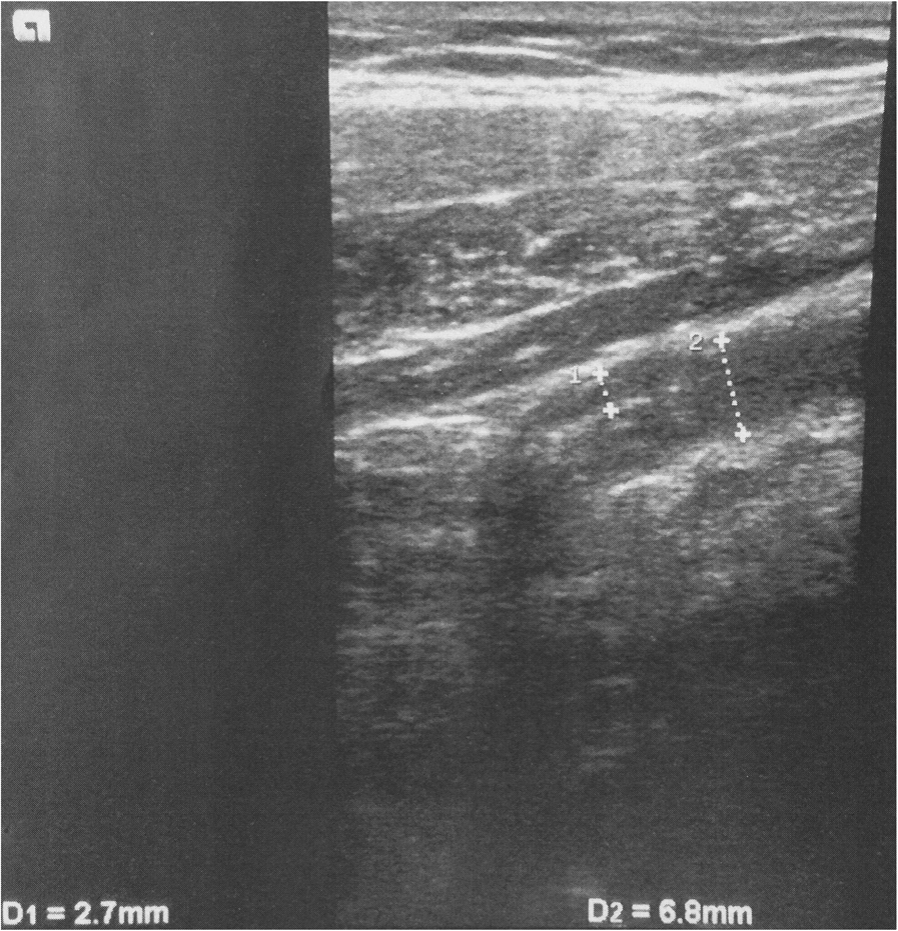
Ultrasonography of the abdomen. An ultrasound-triggered suspicion of acute appendicitis was based on the free liquid around the terminal ileum, the intestinal cockade sign and the increase of the wall thickness of the proximal appendix to 3 mm. D1 (2.7 mm) ist equal to the increased wall thickness with weak echo signal intensity. D2 (6.8 mm) is equal to the diameter of the inflamed appendix in its proximal part
